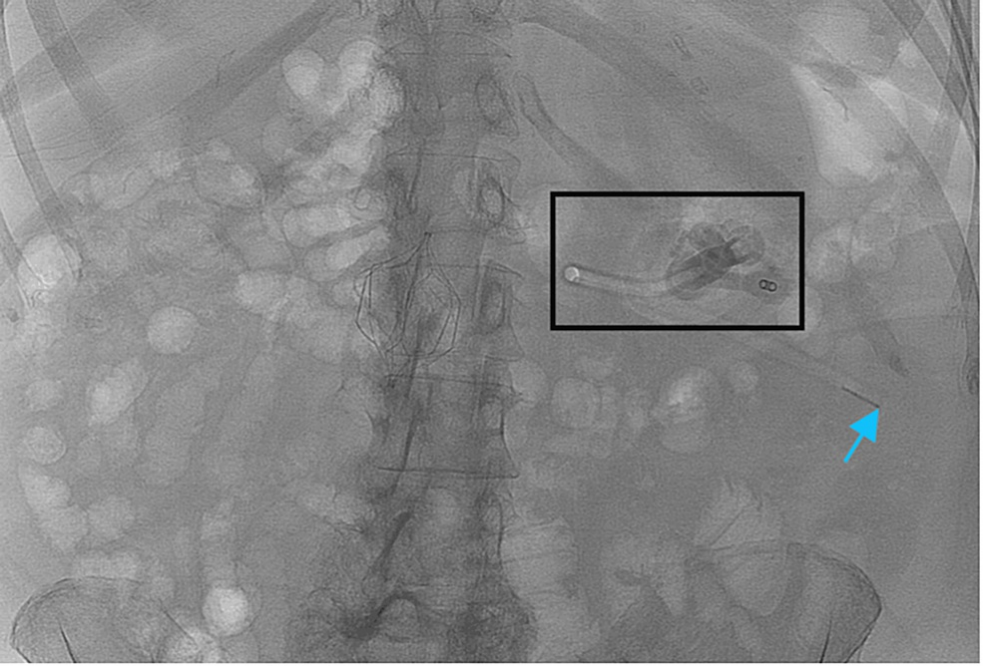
Scout radiograph demonstrates the gastrostomy catheter overlying the left upper quadrant (black box) with the distal tip coursing toward the left lower quadrant (blue arrow).
